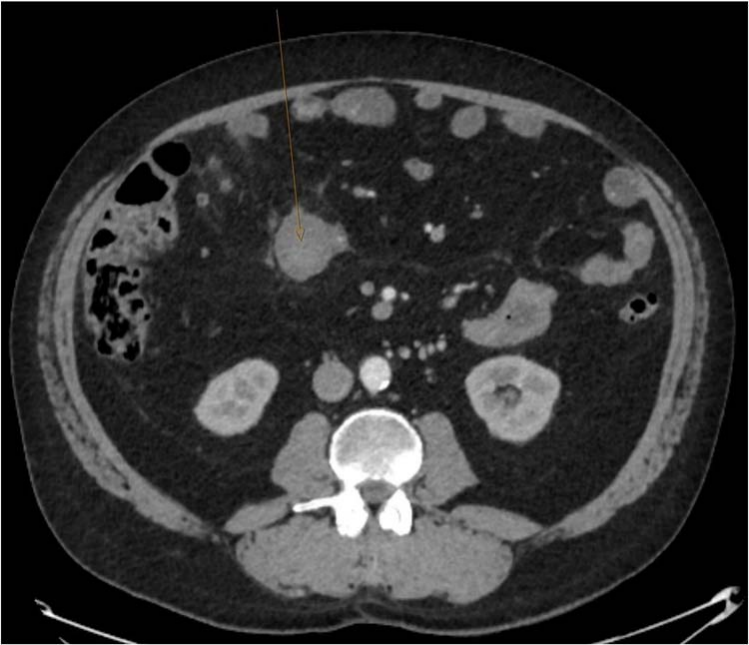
Follow up CT angiogram showing improvement in mesenteric haematoma. Figure 4 is an axial section from the patient’s CT scan performed 4 weeks following discharge. The arrow highlights the patient’s liquefying mesenteric haematoma, which decreased from 104 x 62 mm to 60 x 40 mm. Combined with the improvement in the other areas of intra-abdominal bleeding and the patient’s overall clinical state, this image represents a significant improvement.
